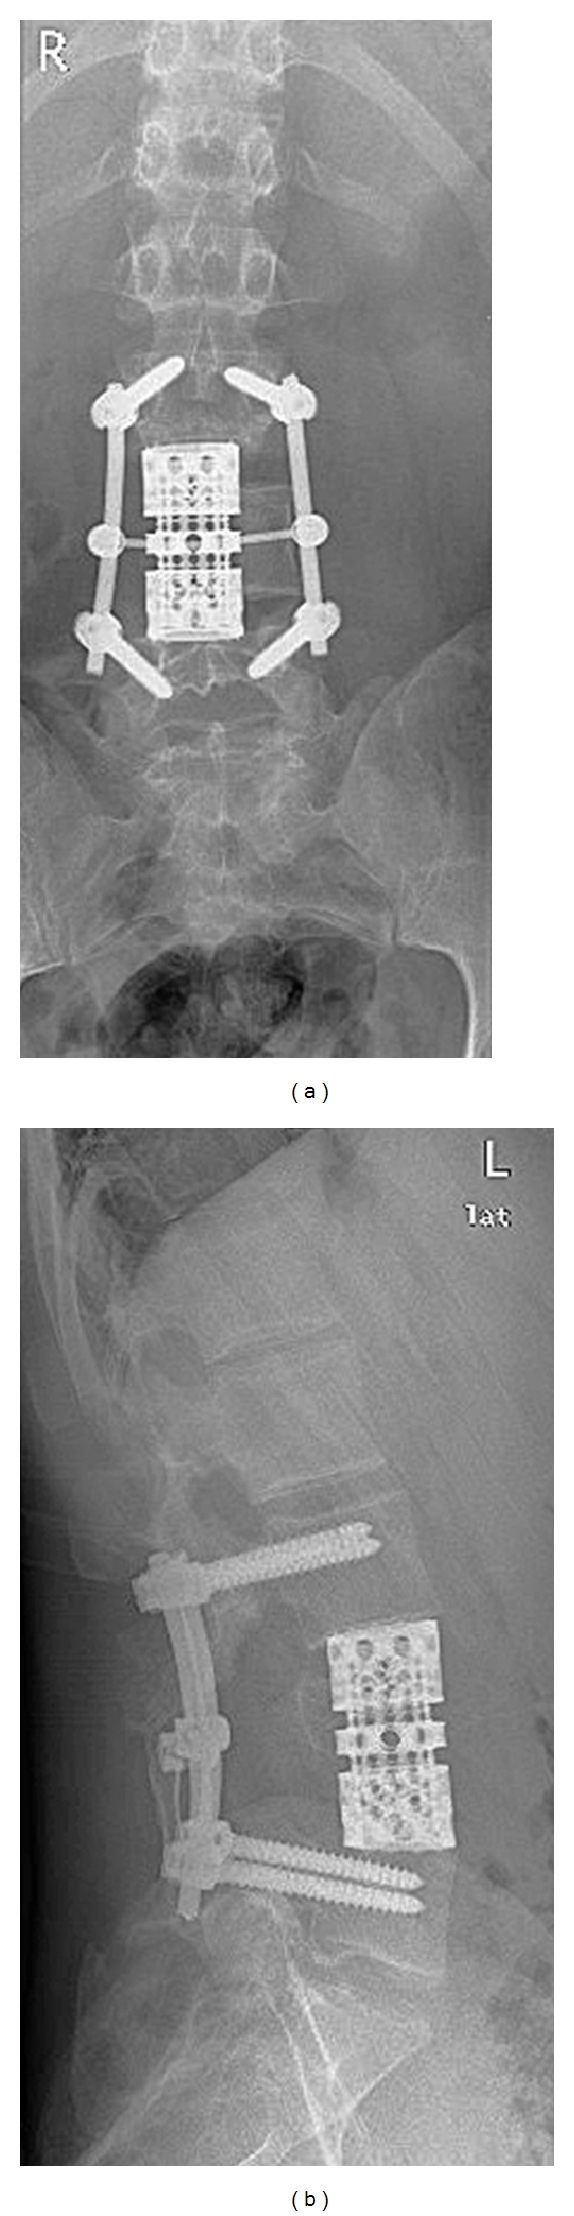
X-ray after dorsoventral spondylectomy. Postoperative X-ray of case 6. Dorsoventral intralesional spondylectomy of L4, dorsal instrumentation from L3 to L5, and titanium cage interposition with bone graft.
